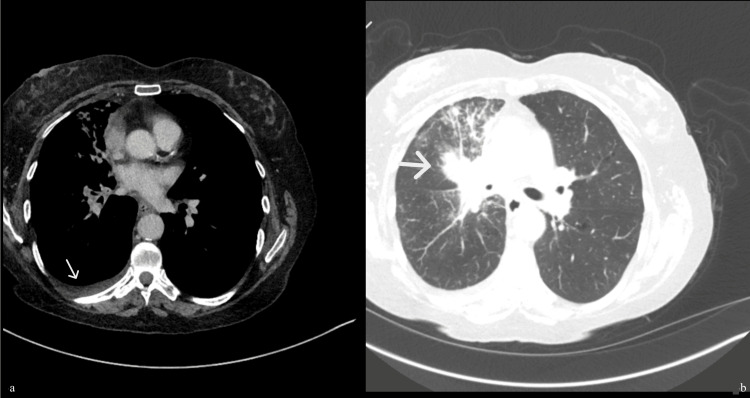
Computed tomography (CT) thorax abdomen and pelvis lung view in the axial section showing right-sided unilateral pleural effusion (a) and right hilar mass (b).
